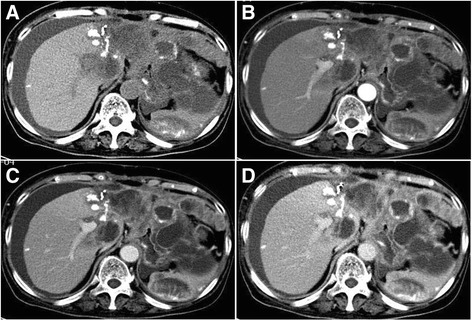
Abdominal CT imaging after chemotherapy. a The left hepatic lobe is absent. Space-occupying lesions are seen throughout the liver, spleen, peritoneum, omentum majus, and mesentery. b–d Extensive massive, nodal-like shadows with heterogeneous enhancement are seen in the liver parenchyma, spleen, peritoneum, omentum majus, and mesentery. There was no clear boundary between the lesion, the incisal edge of left hepatic lobe, and the gastric wall. A low-density filling defect can be observed in the main portal vein
